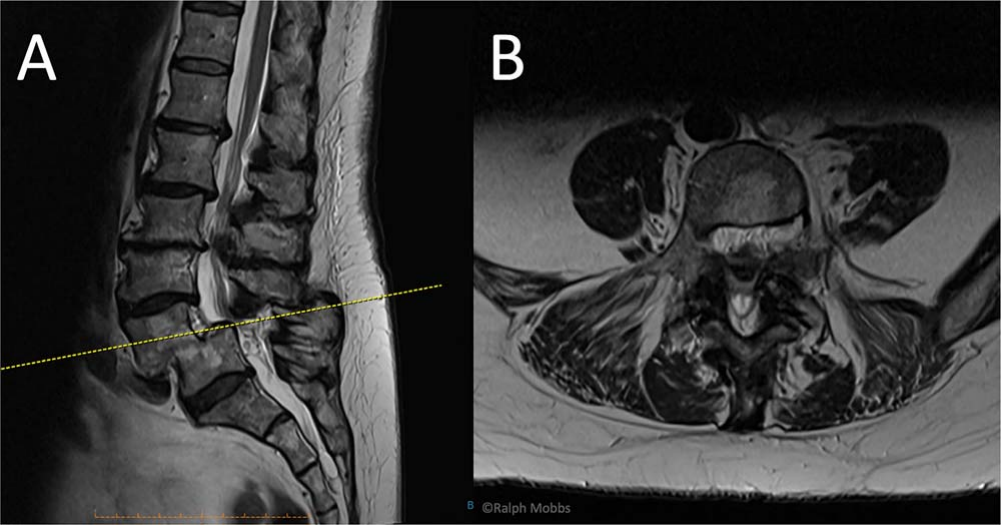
Preoperative MRI of case 2. (A) Sagittal T2-weighted showing L4/L5 spondylolisthesis. (B) Axial T2-weighted.
